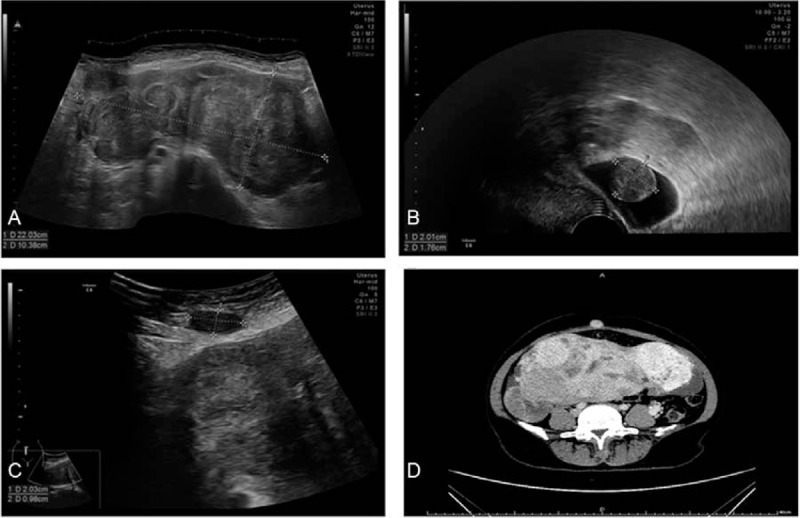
Ultrasound and CT images of Case 1. A, Transvaginal ultrasound showed multiple hypoechoic nodules in uterus, and the uterus lost its normal shape. B, Transvaginal ultrasound showed hypoechoic nodules in uterine rectal area. C, Transabdominal ultrasound showed hypoechoic nodule in paraumbilical abdominal wall. D, Enhanced CT scan revealed irregular soft tissue mass in the lower abdomen and nodules in abdominal wall below the umbilicus.
